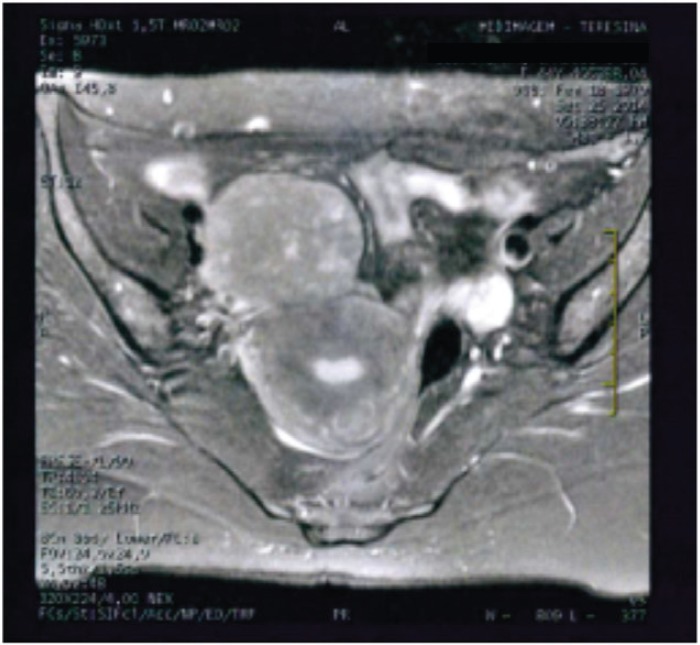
Axial T2-weighted MRI image showing a solid expansile lesion.
